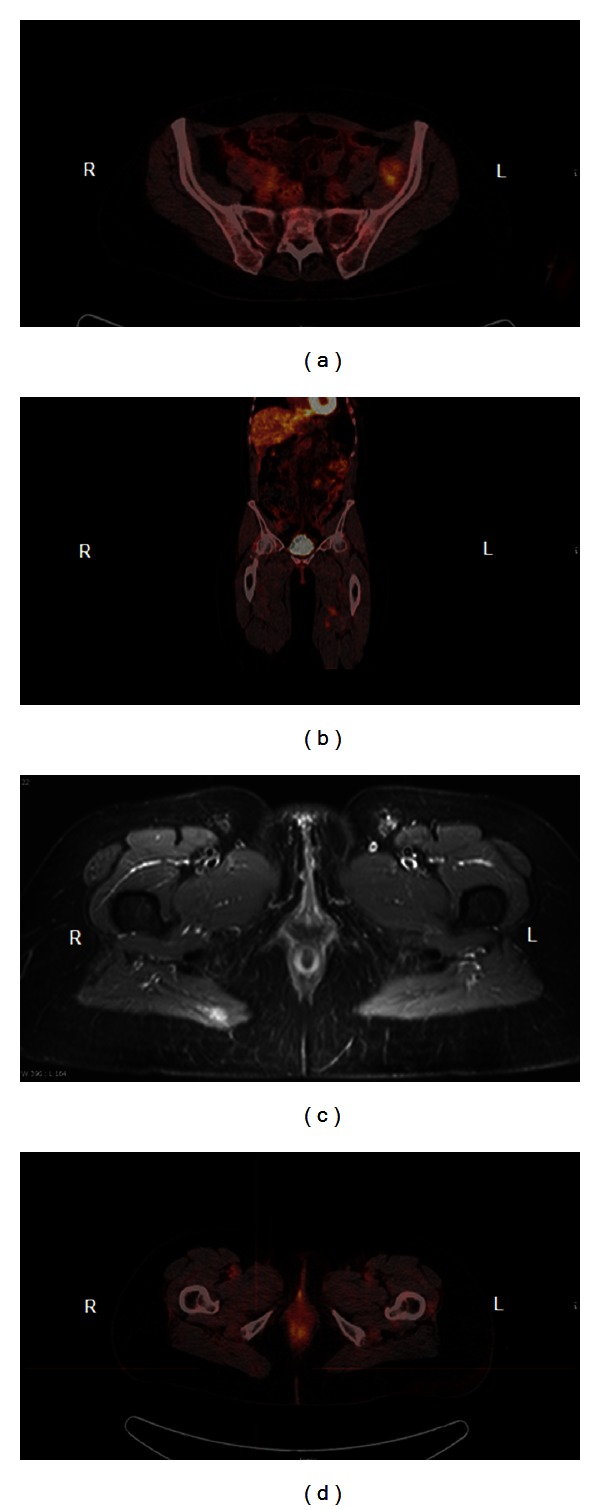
Imaging of the pelvic area in case 14. PET-CT scan showing three positive lesions on the left side: one in the pelvic area (a) and two in the thigh (b). MRI scan showing an additional lesion in the gluteal muscle on the right side (c), not positive on the PET-CT scan (d).
